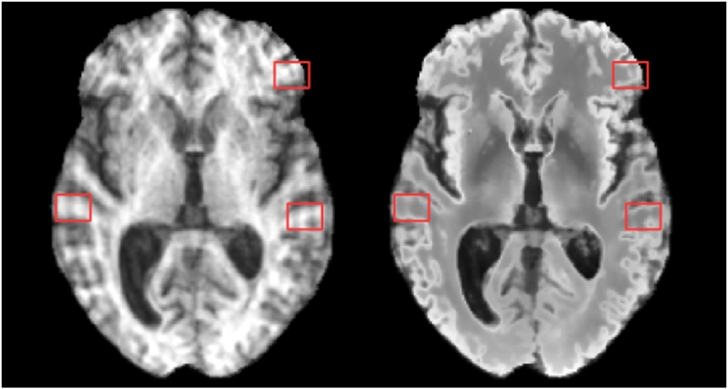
A case where ringing artefacts in a subject's *T*_1_-w image results in errors in the synthesised FLAIR image whereby juxtacortical WM is synthesised as GM in the indicated locations. Left: *T*_1_-w image. Right: Corresponding synthetic healthy FLAIR image.
